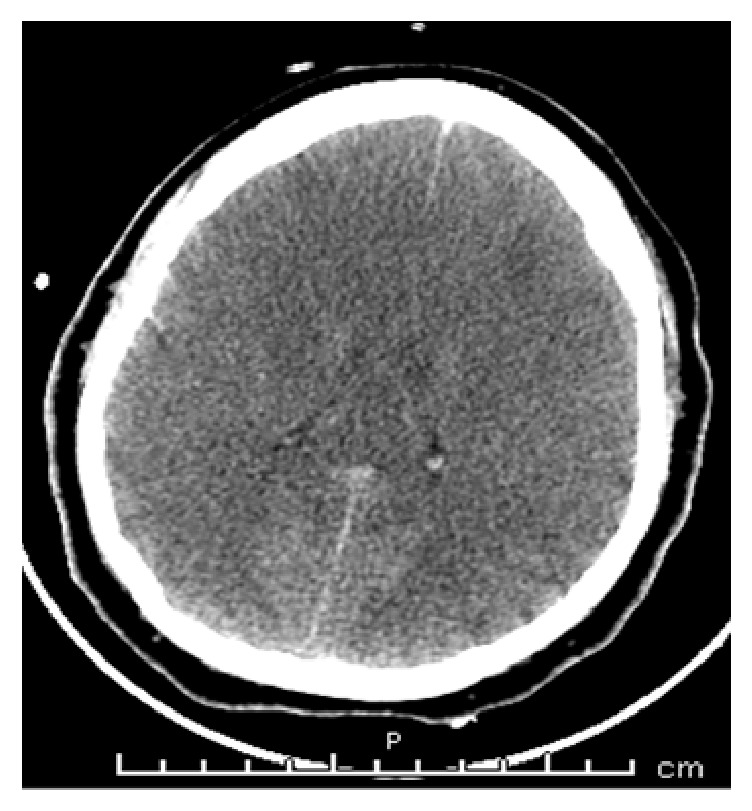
Noncontrast head computed tomography (CT) showing diffuse sulcal effacement and obliterated basal cisterns.
